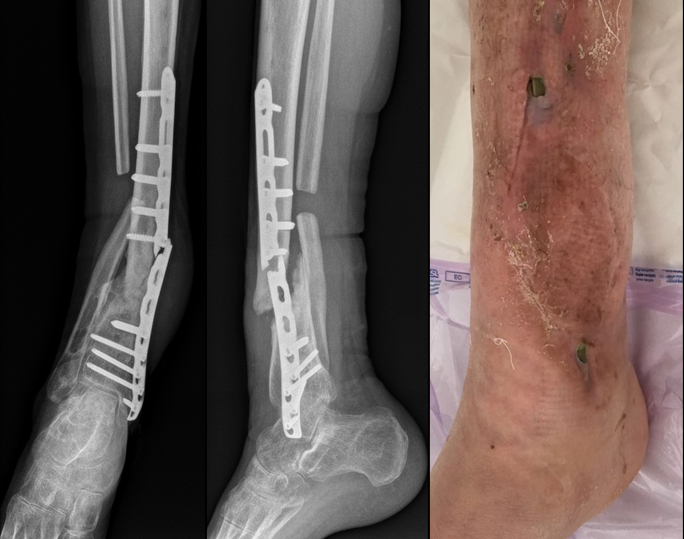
Radiographs and clinical pictures upon admission, in CFRI case
